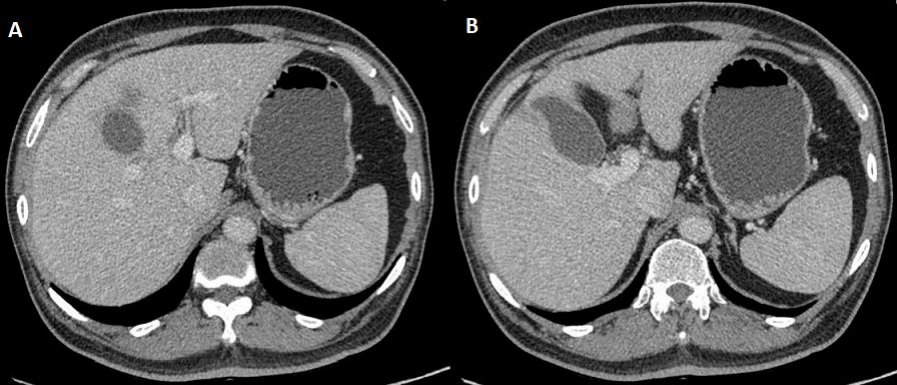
Computerized tomographic (CT) images of the case: A) gall bladder showing diffusely thickened wall and irregularities at fundus; B) surrounding liver shows small area of hypodensity suggestive of gall bladder malignancy with liver metastasis
